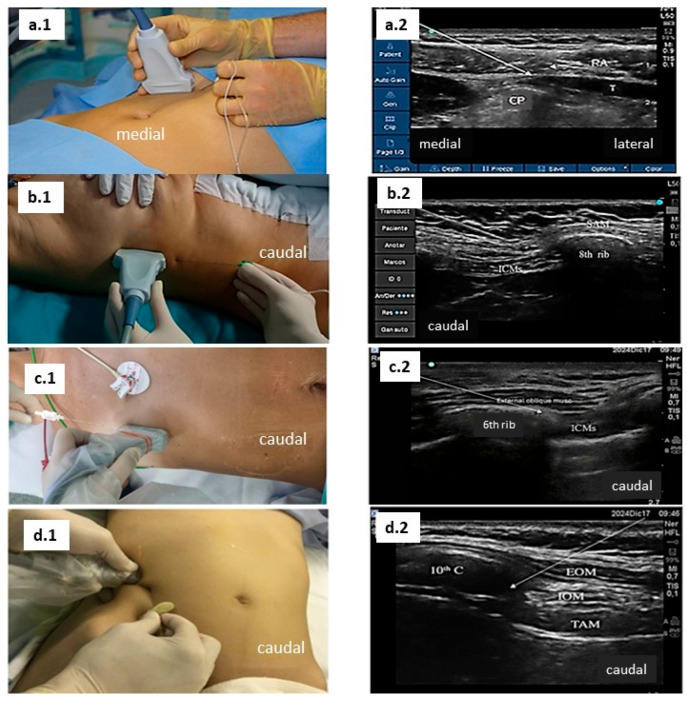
Approaches and ultrasound images: (a.1,a.2). subcostal TAP approach and ultrasound image; (b.1,b.2). SIPB approach and ultrasound image; (c.1,c.2). EIOB approach and ultrasound image; and (d.1,d.2). M-TAPA approach and ultrasound image.
